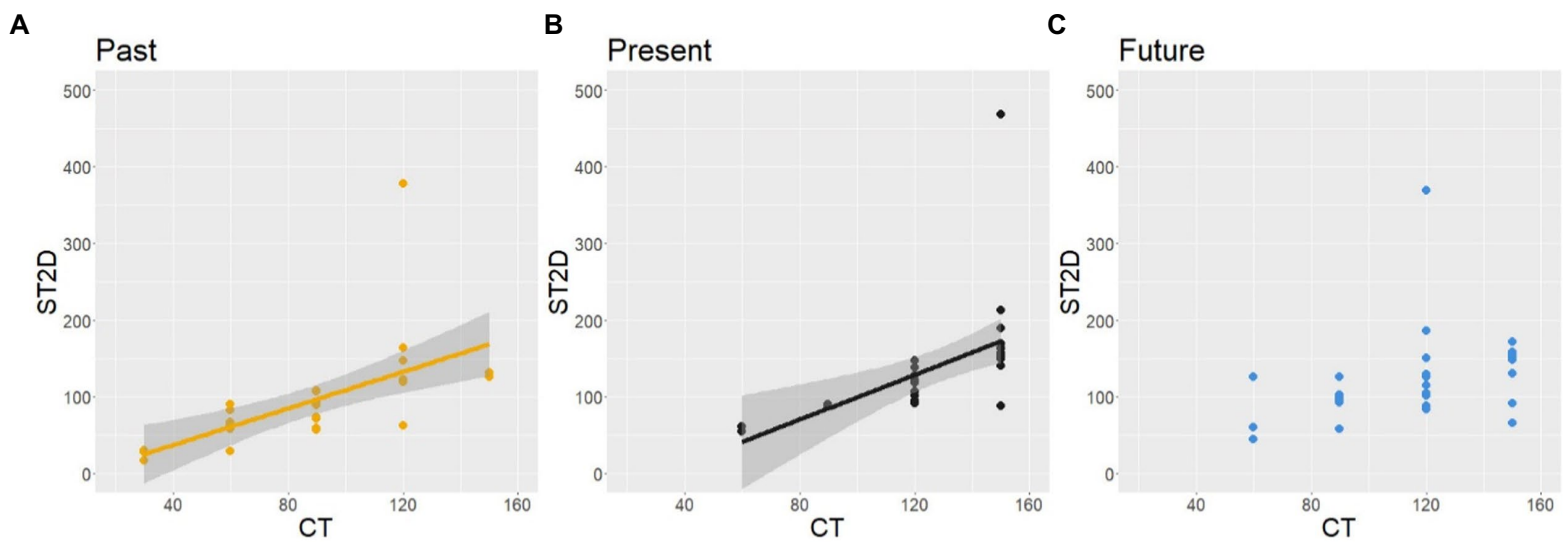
Sizes of spheres in ST on the screen as a function of the sizes of circles in CT. Each dot expresses a participant’s results for the past (A), present (B), and future (C). The gray areas surrounding the regression lines indicate the confidence interval.
